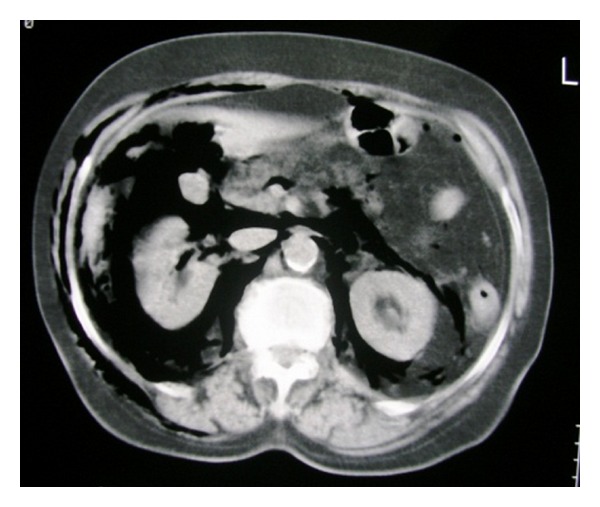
Transverse CT image of the upper abdomen demonstrating subcutaneous emphysema and pneumoperitoneum. Free air surrounds both kidneys and overlaps both great vessels.
